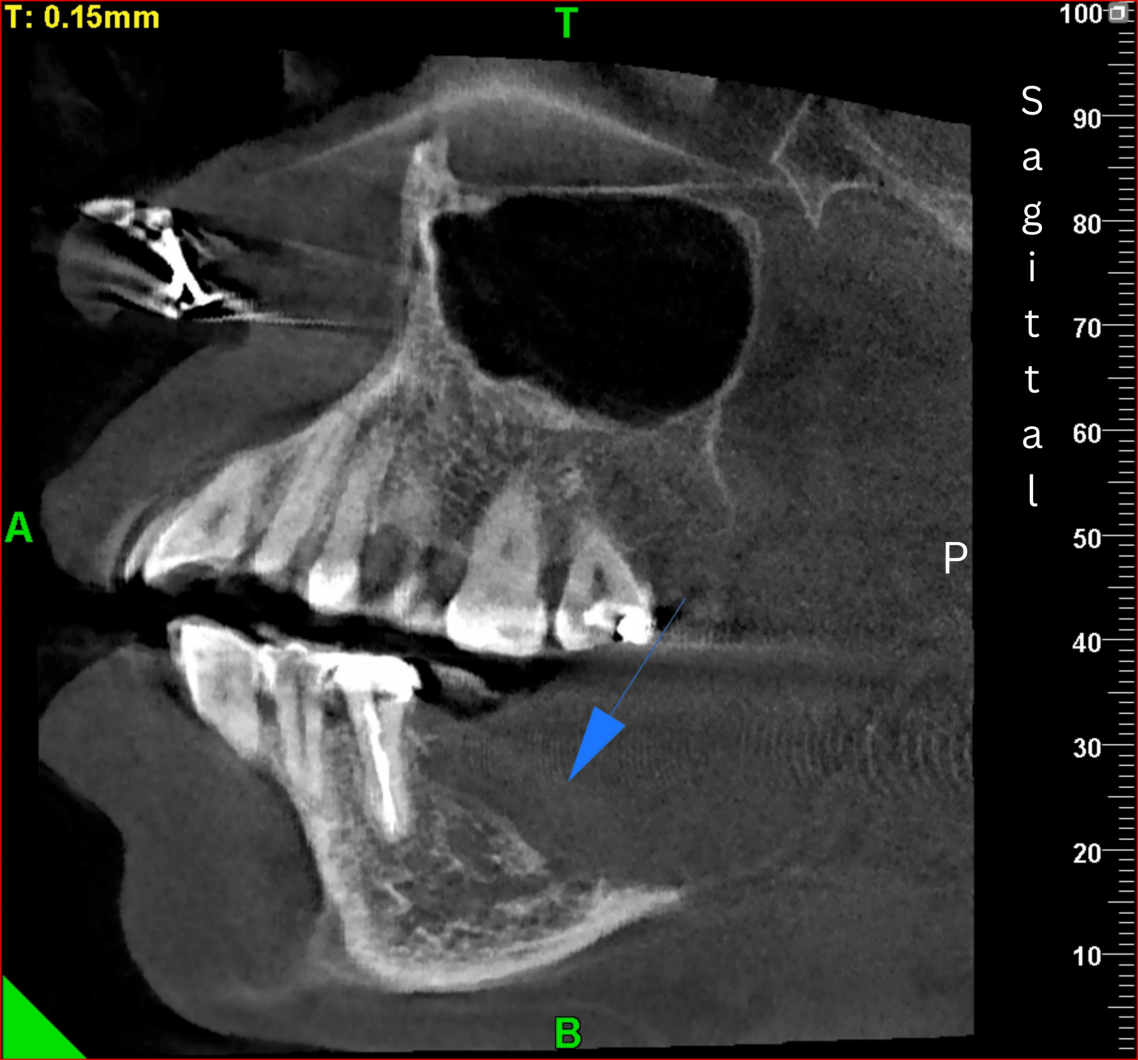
CBCT: sagittal section. The arrow demonstrates the involvement of the body and ramus of the right posterior mandible with destruction of cortical bone and alteration of the surrounding trabecular pattern. CBCT, cone-beam computed tomography
